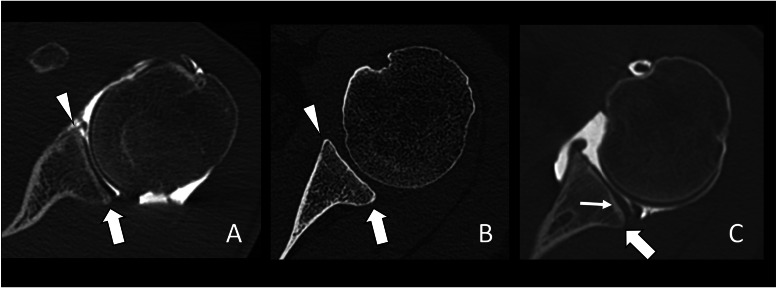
Assessment of the morphology of the posterior glenoid rim on axial CT images. A) 31-year-old male with a symmetric morphology between the anterior (arrowhead) and posterior (arrow) glenoid articular rims evocative of a normal glenoid morphology. B) 51-year-old male with a mild truncation of the posterior glenoid rim (arrow) compared to the anterior rim (arrowhead) evocative of mild glenoid dysplasia. C) 30-year-old female with moderate truncation of the posterior glenoid rim (arrow) with a posterior tilt of the glenoid surface (thin arrow) evocative of a mild glenoid dysplasia.
